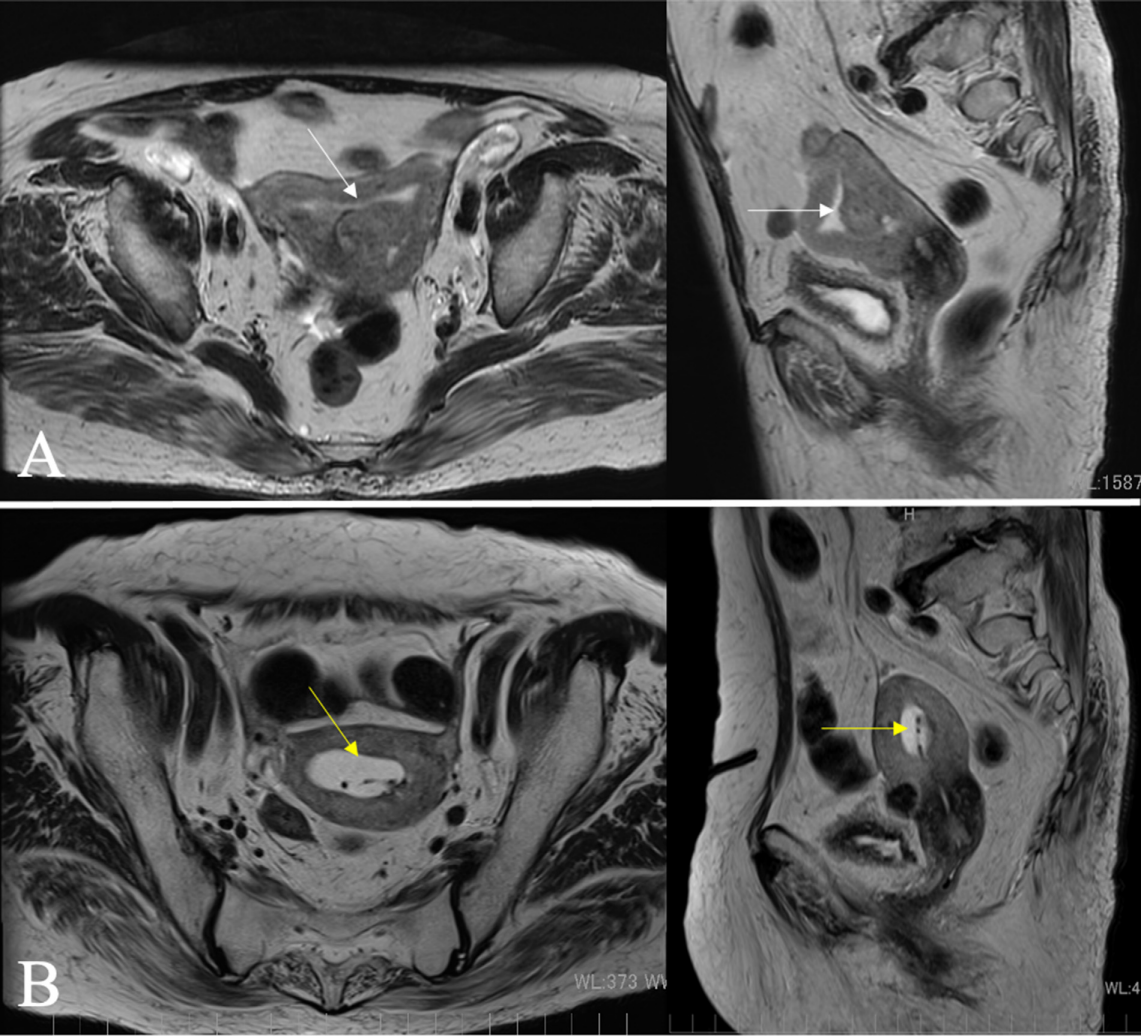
(a) Pretreatment magnetic resonance imaging (MRI) results revealed a tumor in the posterior wall of the uterine body (white arrow). (b) Pelvic MRI revealed a complete response for the tumor (yellow arrow) at 2 months after radiation therapy (the intrauterine device remained in place).
